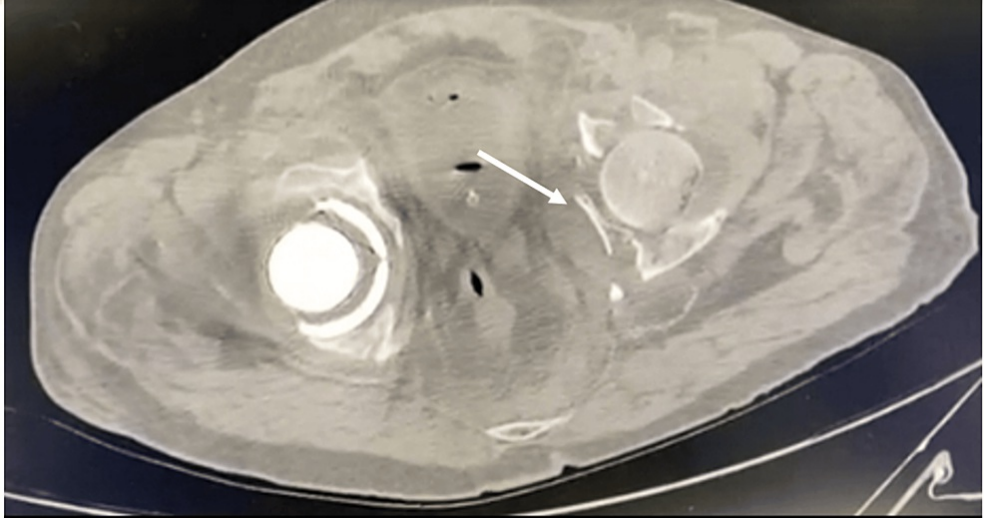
Axial cut CT scan of the pelvis showing left acetabulum fracture with displacement. CT: Computed tomography
